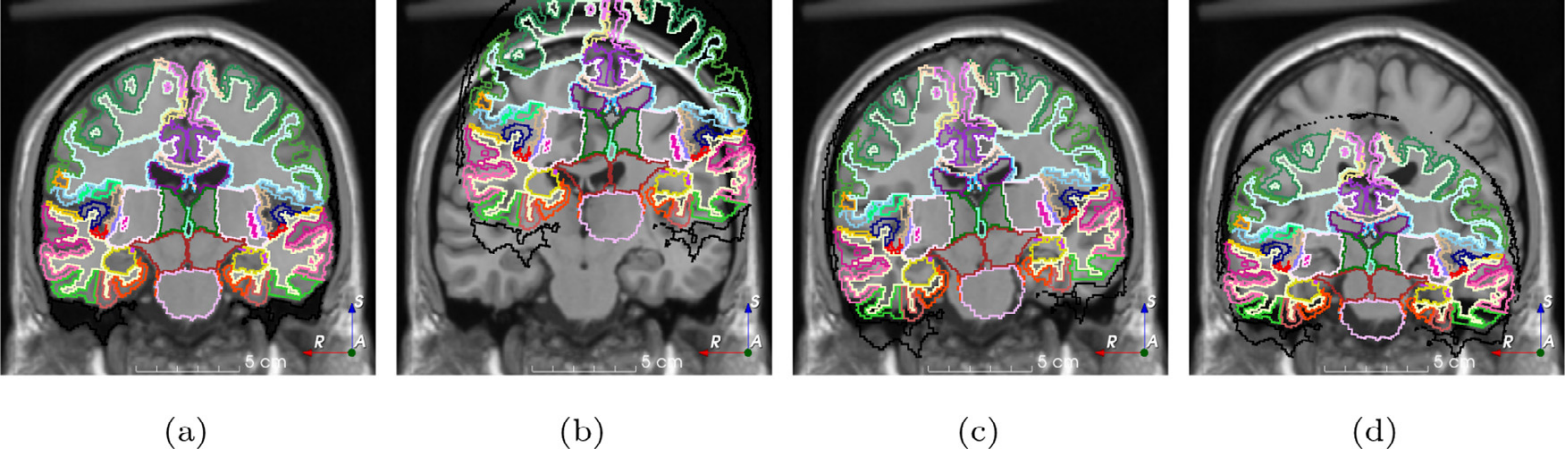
Demonstration of the importance of spatial metadata in medical image processing. The size of both the MRI and the segmentation is 181×181. When spatial metadata is taken into account (a), images are correctly superimposed (only the borders of each region are shown for clarity purposes). Images are incorrectly superimposed if (b) origin, (c) orientation or (d) spacing are ignored.
